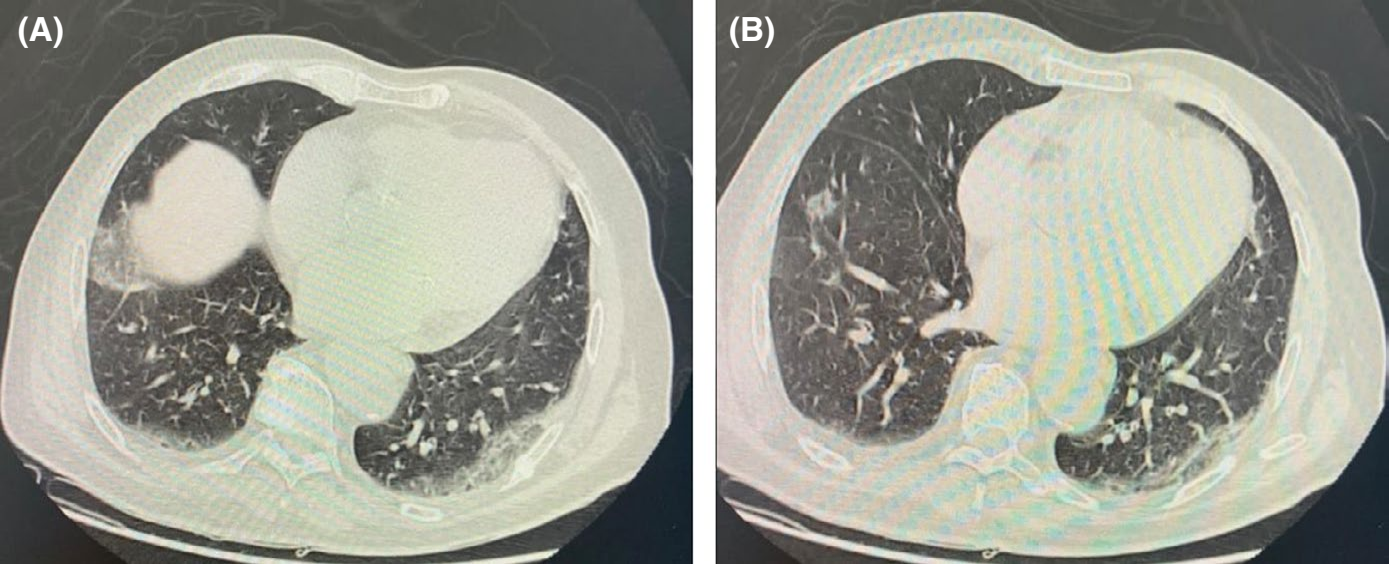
Chest CT scan showing asymmetric bilateral interstitial pneumonia CORADS 5 suggesting a COVID‐19 type infection (A, B)
